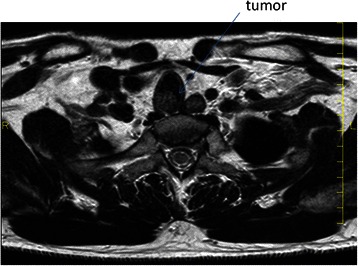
MRI showed a smooth, well-circumscribed nodular shadow was revealed in the posterior wall of the thoracic trachea. The arrow shows a neoplasm in the trachea
